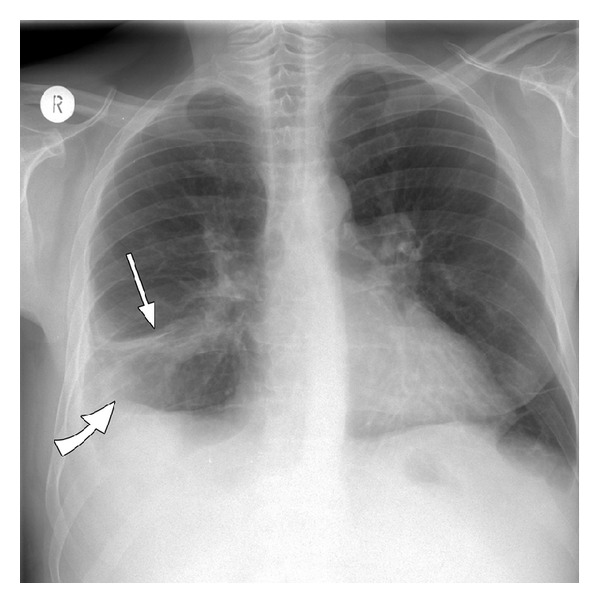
Chest X-ray showing an area of consolidation in the right lower field (straight arrow) with pleural effusion (bent arrow).
